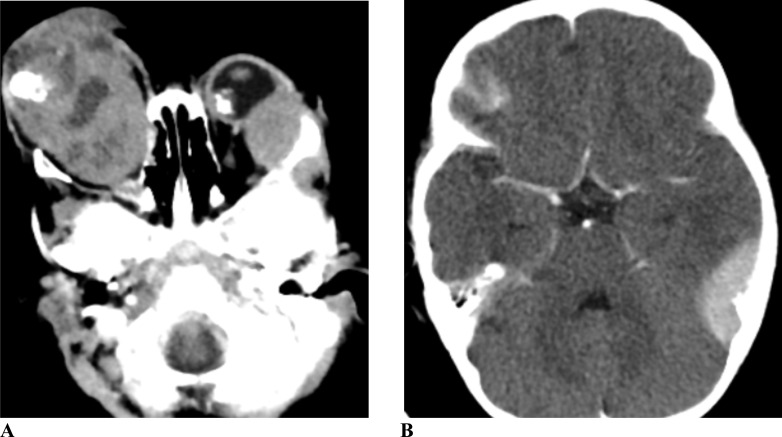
Post-contrast axial brain CT of a 2 year-old female with bilateral retinoblastoma showing heterogeneously enhancing mass replacing the right globe and involving all the subspaces of the orbit having internal areas of dense calcification. There is also focal lesion in the posteromedial wall of the left eye globe. There are homogenously enhancing lesions involving extraconal space in the left orbit (A) and extra-axial space in the left occipitotemporal convexity (B) suggestive of intracranial metastasis.
